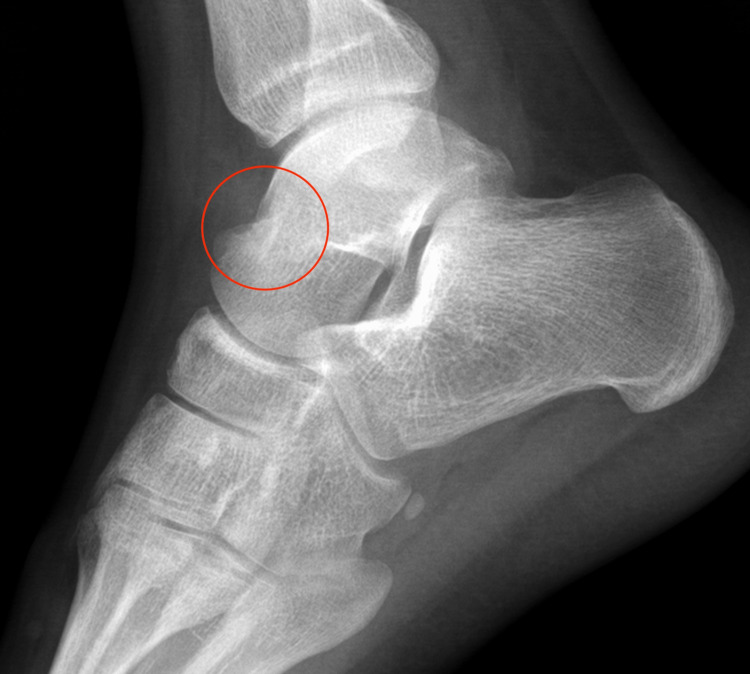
The patient's review with plain radiographs a year after the onset of his symptoms. The presence of a nidus is evident.
